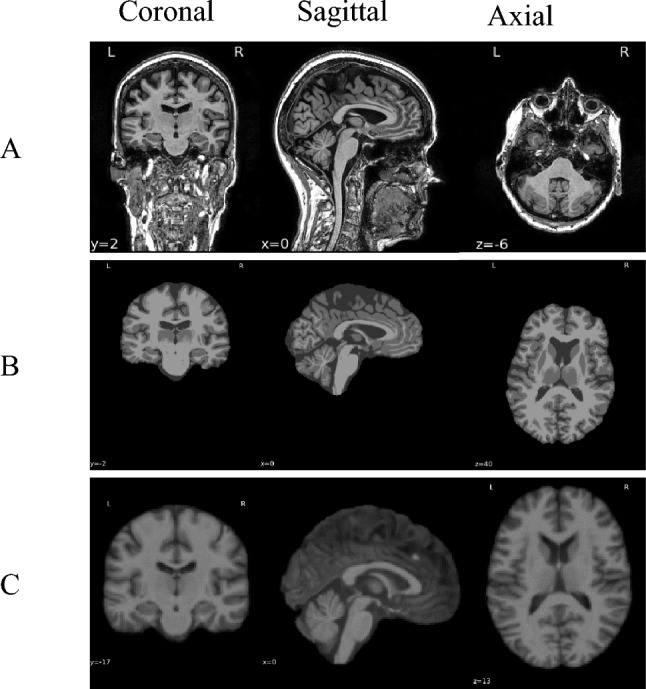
A sample of an MRI slice in three planes (coronal, sagittal, and axial). (A) Original MRI; (B) segmented anatomical image with the skull removed; and (C) segmented anatomical scan warped to MNI space.
